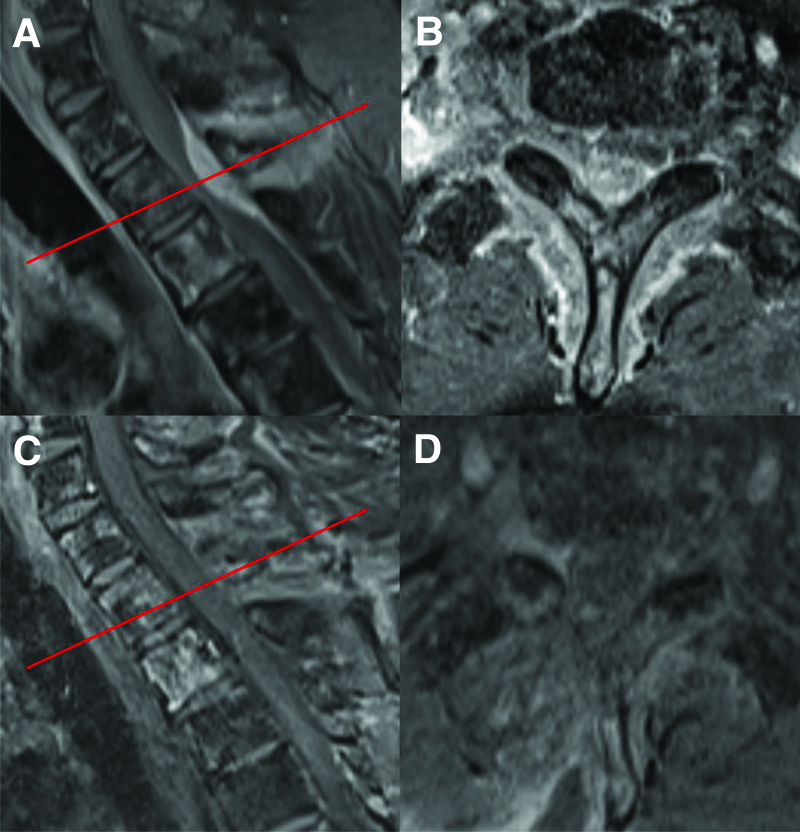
A 59-year-old man diagnosed with prostate cancer presented to the clinic with gait disturbance and pain and tingling sensation in both arms. Preoperative sagittal (A) and axial (B) MRI show a metastatic epidural mass, which compresses the spinal cord at the T1–2 level. As the facet joint and pedicles are intact, DS without fixation is performed. Follow-up sagittal (C) and axial (D) MRI show total removal of the tumor. Each sagittal level of the axial images is indicated by red lines. DS = decompression surgery, MRI = magnetic resonance image.
